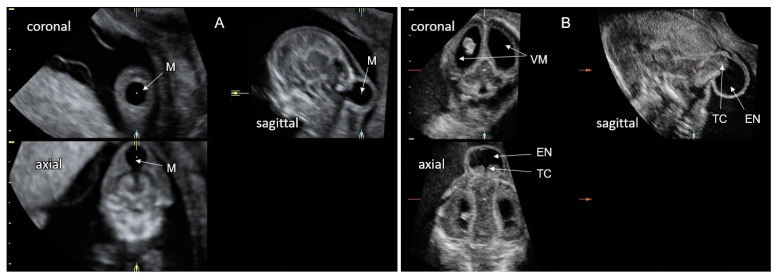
Fetal posterior cephaloceles at the late first and early second trimesters. Legend: (A,B) Three-dimensional sonographic multiplanar reconstruction images demonstrating the coronal, mid-sagittal, and axial head planes of two fetuses with posterior cephalocele. (A) An occipitocervical meningocele (M) without neural tissue inside the lesion at 12 gestational weeks. (B) Occipital encephalocele (EN), containing herniated tectum and cerebellum (TC) at 17gestational weeks; note the dilated lateral ventricles (VM).
